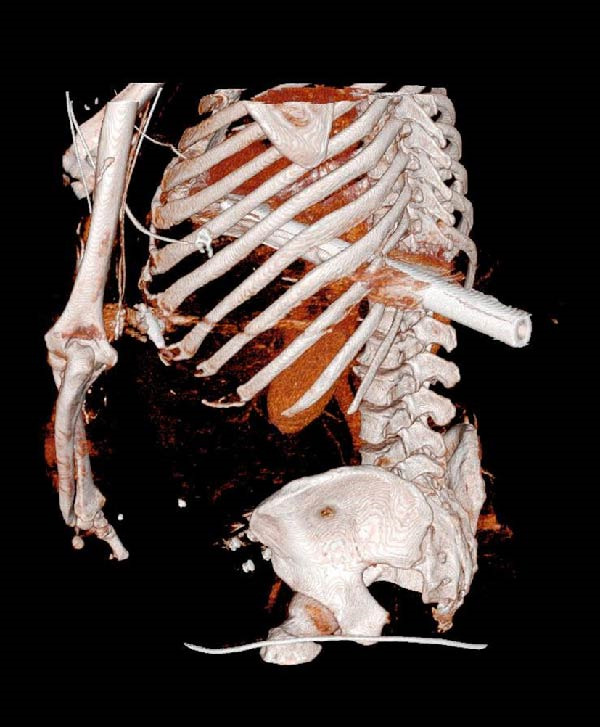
Three‐dimensional computed tomography reconstruction.
